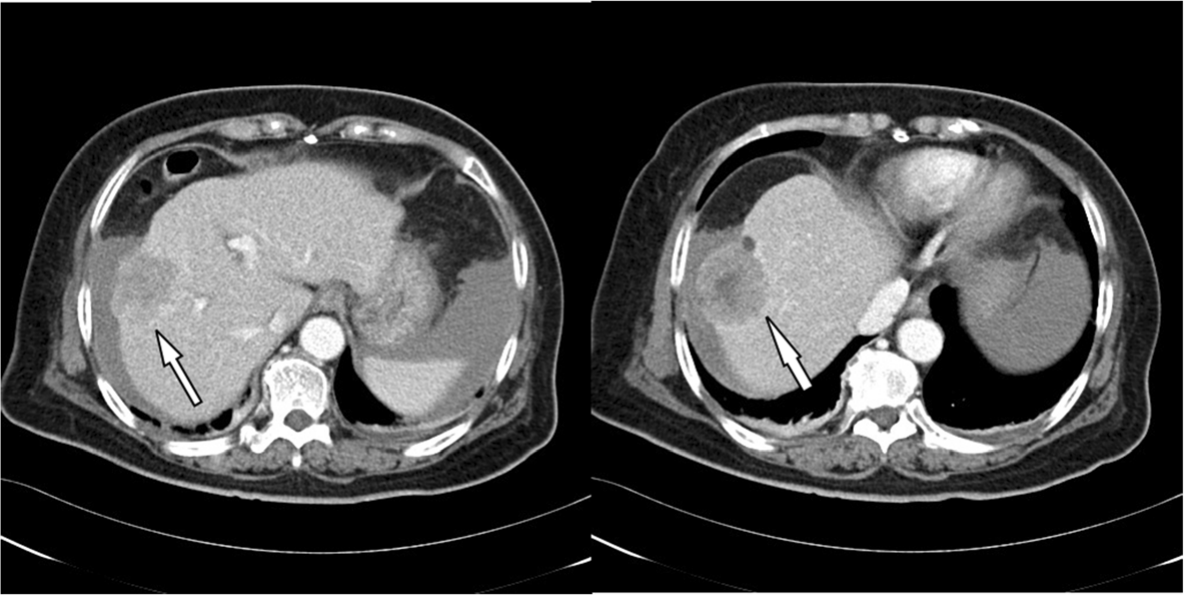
Tri-phasic abdominal CT revealing a large tumor (4.8 cm in diameter) over segment 8 of right lobe compatible with hepatocellular carcinoma (arrow)
